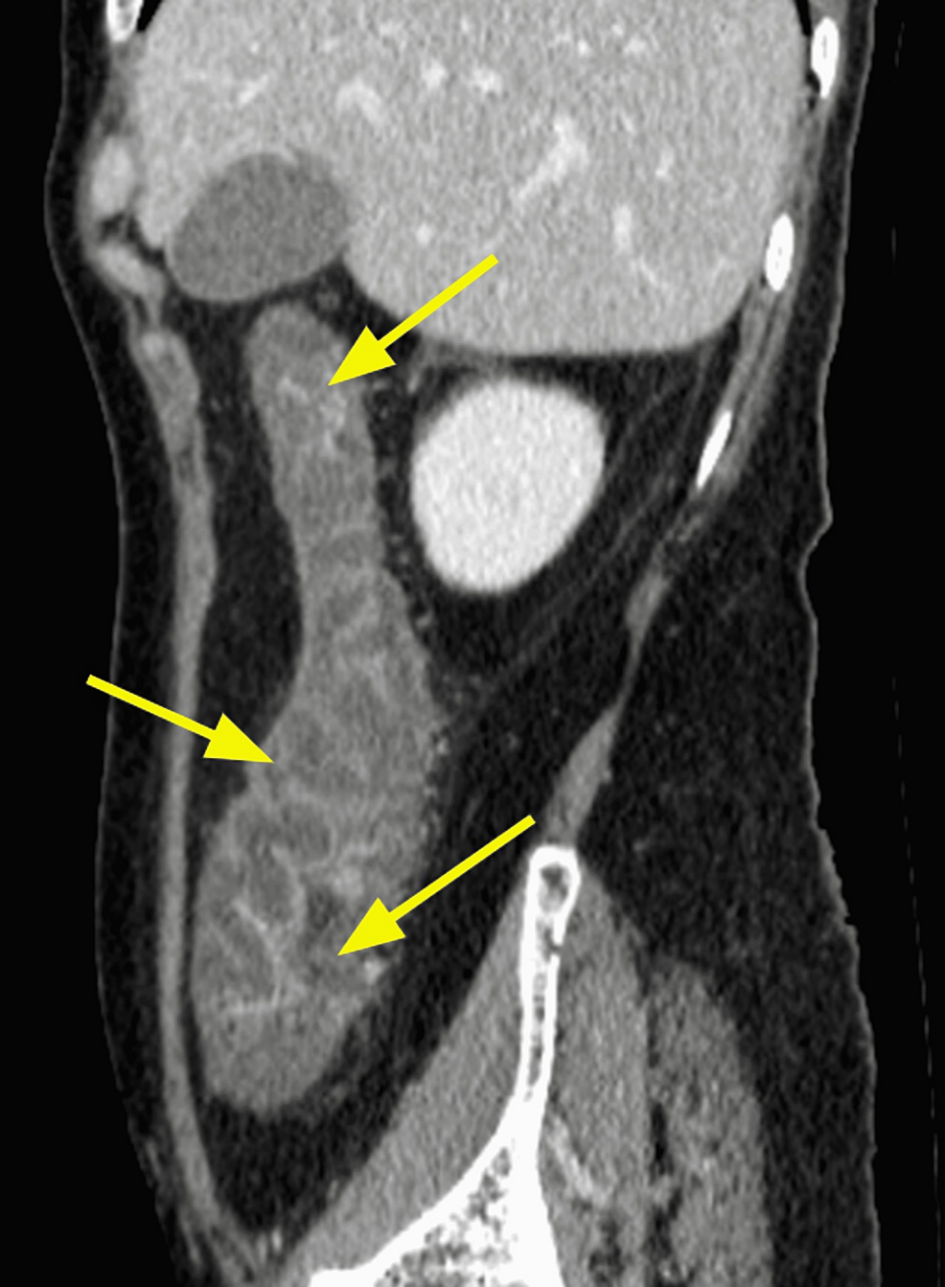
Contrast-enhanced CT for Case 3 (sagittal plane) demonstrating thickening of colonic wall and infiltration of the pericolic fat forming the "accordion sign" (arrows)
